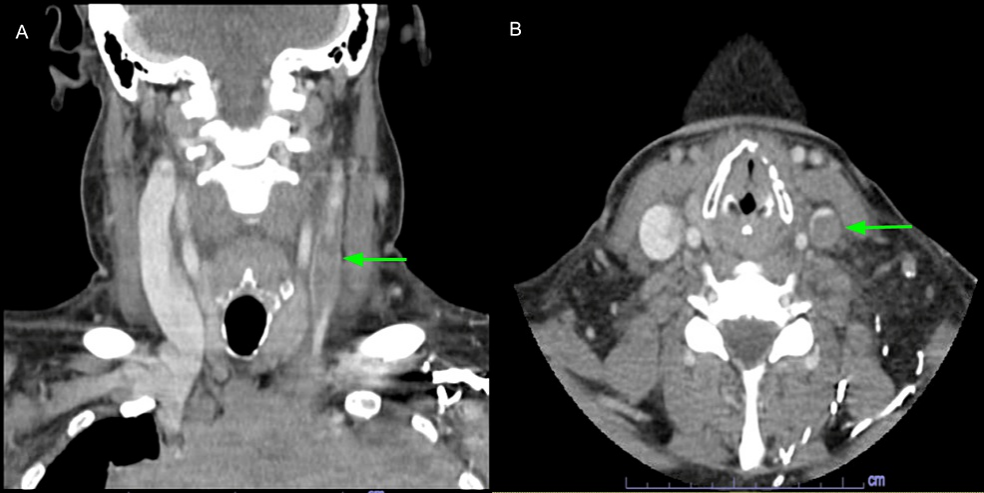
CT head and neck with contrast (A: sagittal view; B: axial view) showed lower left internal jugular vein expansion with internal hypodensity consistent with thrombosis (green arrows).
